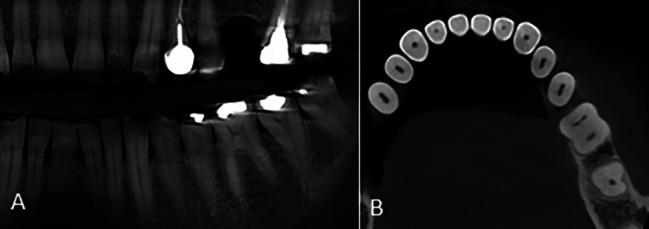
Mandibular second molar tooth with (A) type 5 morphology on the panoramic-like image and (B) single-root pattern on the axial CBCT section
